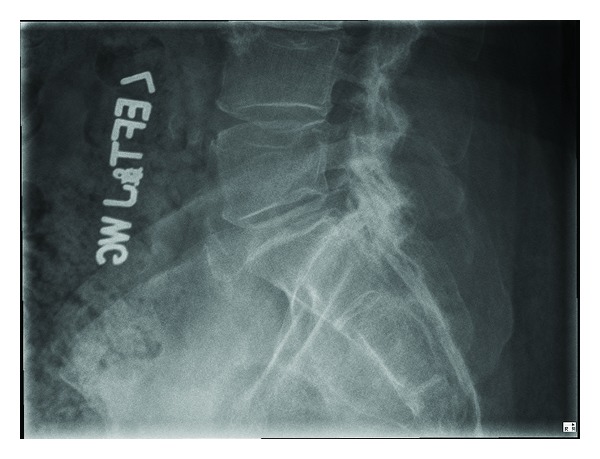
Lateral lumbar X-ray normal lumbosacral alignment and anatomy.
